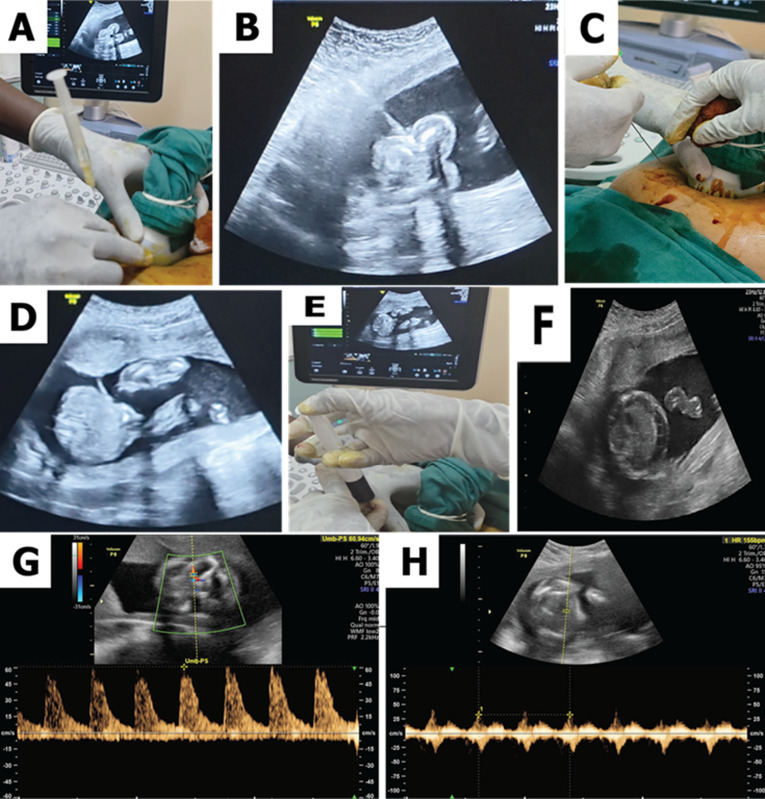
ultrasound-guided intrauterine transfusion at 21+4 weeks' gestation; A,B) fetal sedation with intramuscular atracurium; C-E) intrauterine intravascular transfusion under ultrasound guidance; F) post-transfusion wheal around the fetal liver; G-H) confirmation of fetal viability post-transfusion
